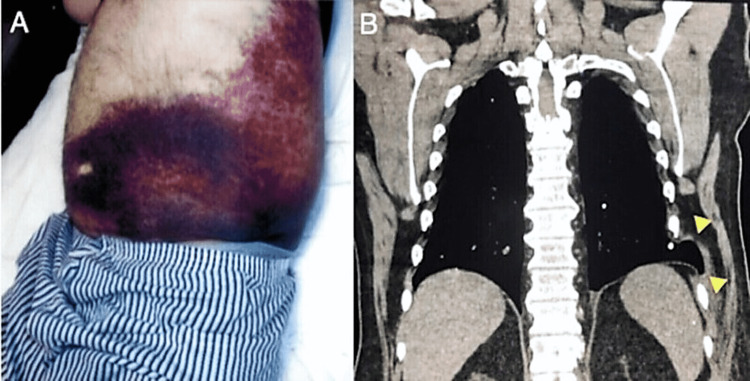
Clinical presentation of the patient (A) Ecchymosis on the left hypochondrium and the left iliac region, extending over the lower abdomen area. (B) Chest CT scan showing a left lateral hernia extending through the intercostal space between the eighth and ninth ribs (yellow arrowheads).
